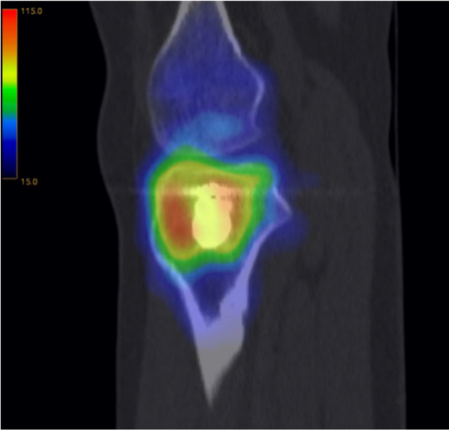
A sagittal view of a SPECT/CT corresponding to Fig. 6 is shown
